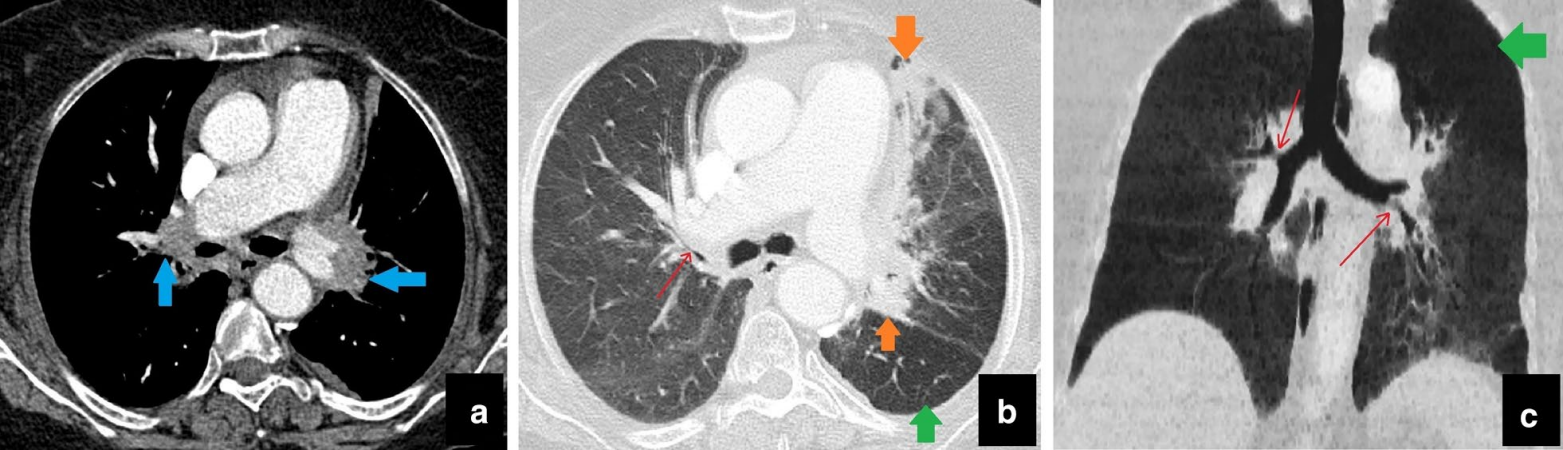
History-related (type 8) error. A 75-year-old female patient presented at the Chest Clinic with a progressive cough. Thorax CT was reported as "bilateral residual tumoral soft tissue (blue arrows, a) and parenchymal fibroatelectatic changes due to radiotherapy (orange arrows, b)." The referrer gave feedback to the radiologist about "the negative history of malignancy and radiotherapy," and intensive biomass exposure was mentioned. The CT was re-evaluated by the radiologist. Paramediastinal parenchymal changes (blue and orange arrows, a, b) were interpreted as a result of “bronchial anthracofibrosis” after combining the associated air trapping (green arrows, b, c) and bronchial narrowings (red arrows, b, c). Although the radiologist made an unwarranted assumption about prior malignancy and treatment, the main reason for this reporting error was insufficient clinical information about “biomass exposure.” This case is a good example of “error with no harm” thanks to the effective communication between the radiologist and the clinician via the clinician’s feedback
